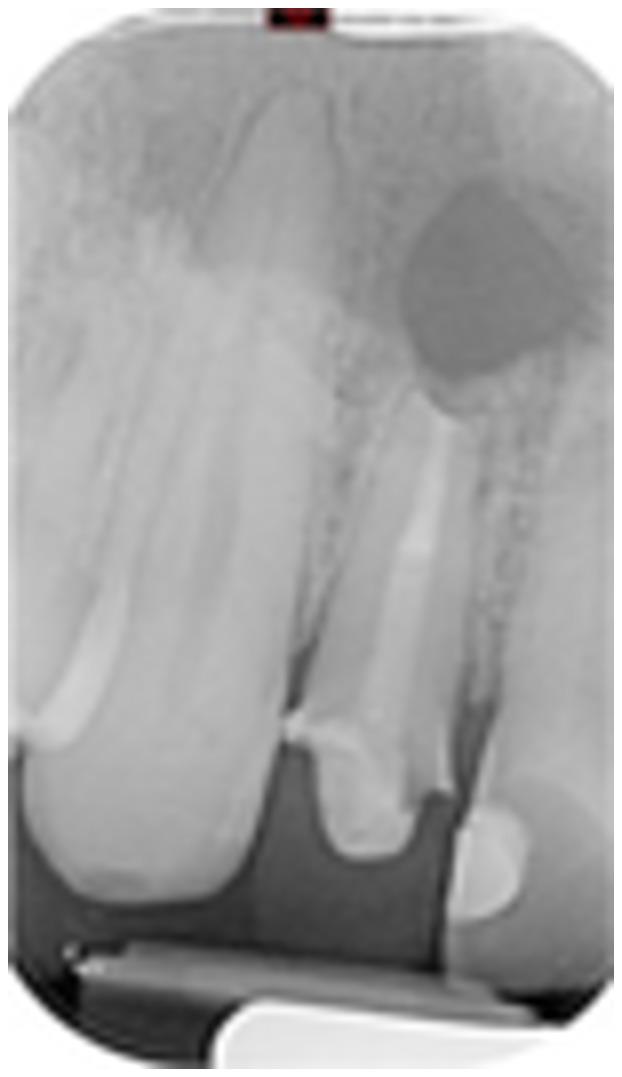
Case 1: The preoperative CBCT and periapical radiograph show an extensive periapical radiolucency extending from teeth 11 to 13. Only tooth 12 contributed to the infection and inflammation; therefore, apical surgery was only performed on tooth 12 following satisfactory nonsurgical root canal treatment. At 6 months, marked healing can be seen, evidenced by a reduction in the periapical radiolucency size, now confined to the apical region of tooth 12 only.
